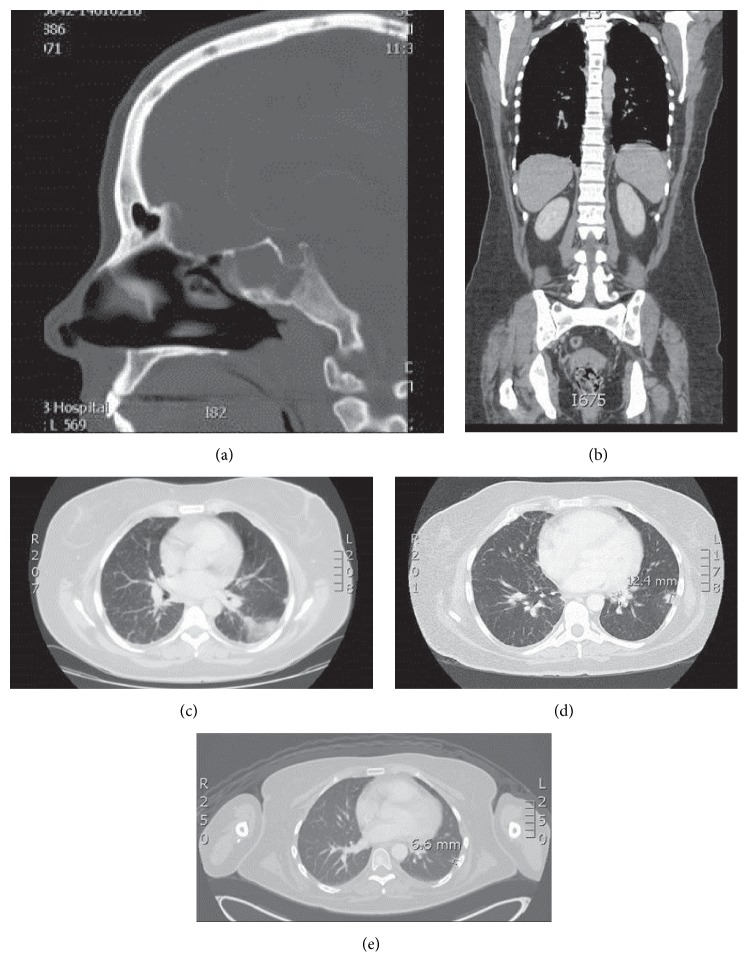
(a) Sagittal view of the sinus on computerized tomography (CT) showing the complete opacification of the right sphenoid sinus which was proven to be ALCL involvement by biopsy. (b) Axial view of CT C/A/P obtained at the time of diagnosis showing the diffuse osseous metastatic spread of disease involving vertebral bodies, pelvis, and humerus. (c) Representative lung field section of the CT C/A/P in (b) showing the numerous subcentimeter nodular densities within the lung parenchyma and along the margins of the fissures and pleural margins. Patient did not have significant lymphadenopathy (not shown). (d) Representative CT lung field performed after the first dose of hyperCVAD without BV showing interval progression of lung disease as well as development of new right hilar adenopathy (not shown). (e) Representative lung field of PET-CT (level approximates that of image (d)) showing resolution on the reticulonodular opacities and decrease in size of the largest subpleural nodule in the L lung. No PET avidity noted. Not shown are persistent diffuse lytic lesions that are not PET avid.
